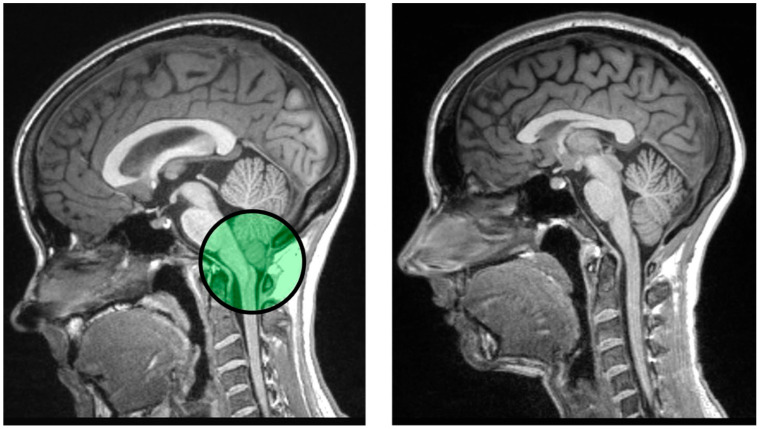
MRI presentation of Chiari Malformation Type I and a healthy control. Note. T1-weighted structural midsagittal images are provided. The CMI patient image is presented to the left. A healthy control is presented to the right for reference. The green area highlights the displacement of the cerebellar tonsils below the foramen magnum. This displacement acts as the pathognomonic radiological feature of CMI.
